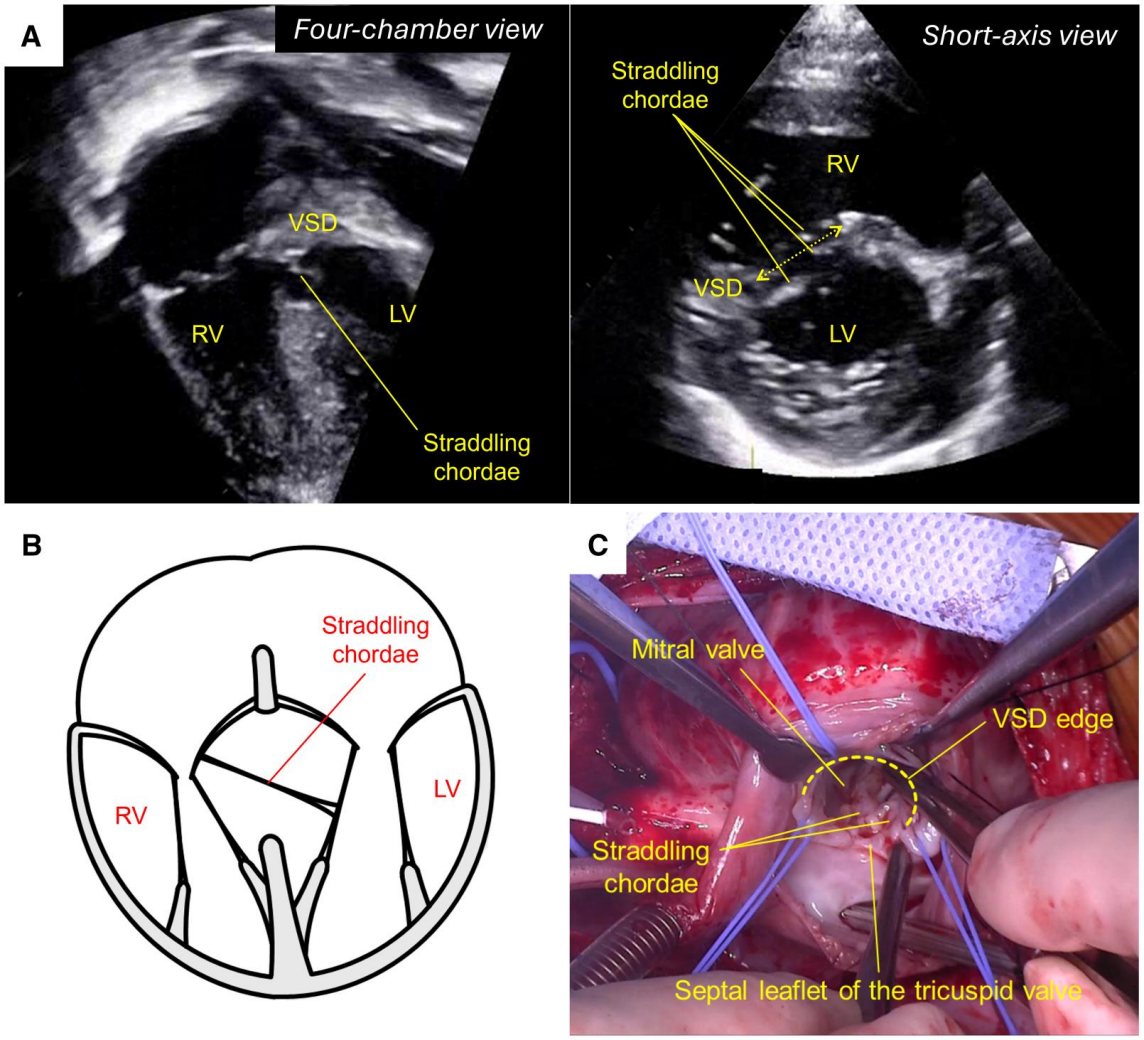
(A) Preoperative Echocardiography, (B) Straddling Chordae, and (C) Intraoperative Image. Abbreviations: LV, left ventricle; RV, right ventricle; VSD, ventricular septal defect
